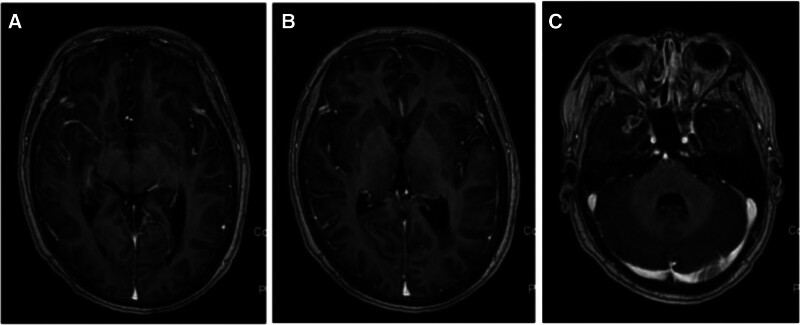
Follow-up visit in October 2023. Compared with the confirmed diagnosis, the patient’s brain MRI showed no significant deterioration. The left temporal lobe enhancement disappeared, and new right temporal lobe enhancement was found. MRI = magnetic resonance imaging.
